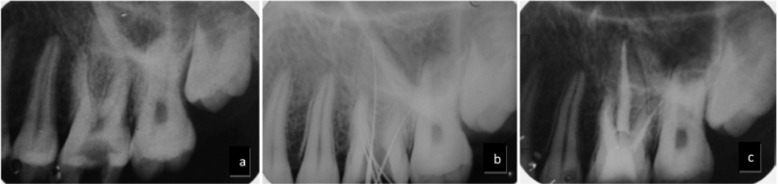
IOPA radiographs of the maxillary first molar. a. Pre-operative radiographs; b. Shows the working length radiograph ; c. Post-obturation radiograph.
